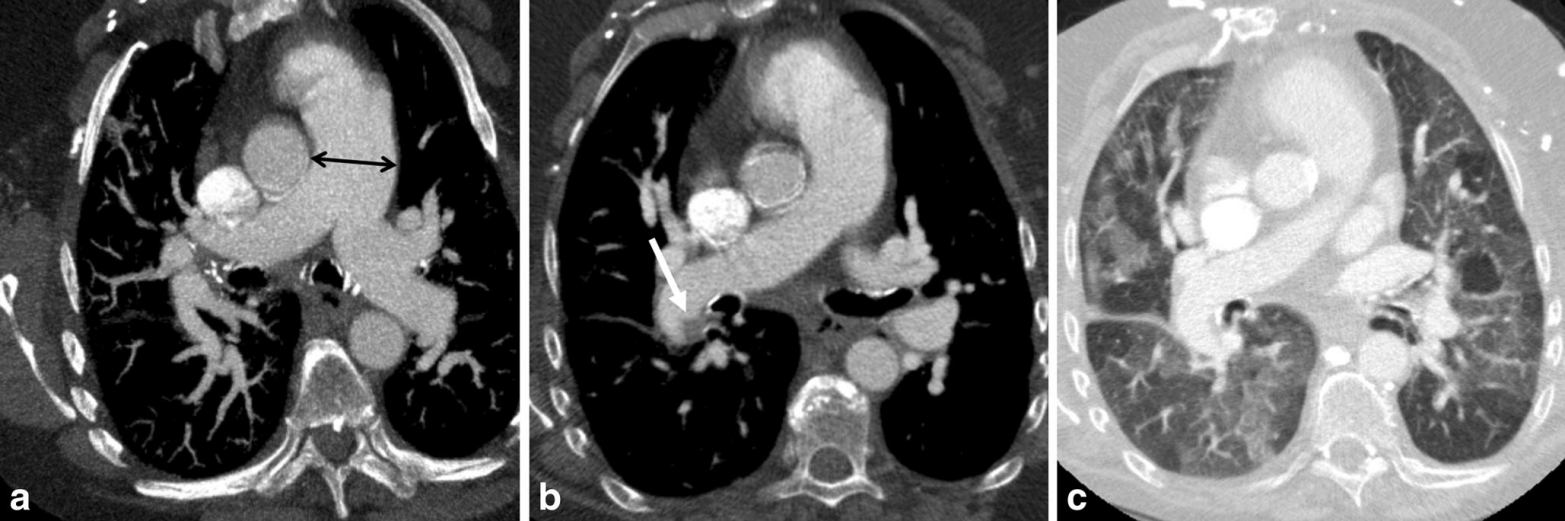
A 62-year-old male presented to the ED with exertional chest pain and progressive dyspnoea. He is known with systemic lupus erythematosus. (a) The CT images show a dilatation of the pulmonary trunk and the left and right pulmonary arteries (arrow), (b) with eccentric thrombotic material in the right pulmonary artery (arrow), and (c) mosaic attenuation consistent with chronic thromboembolic pulmonary hypertension
